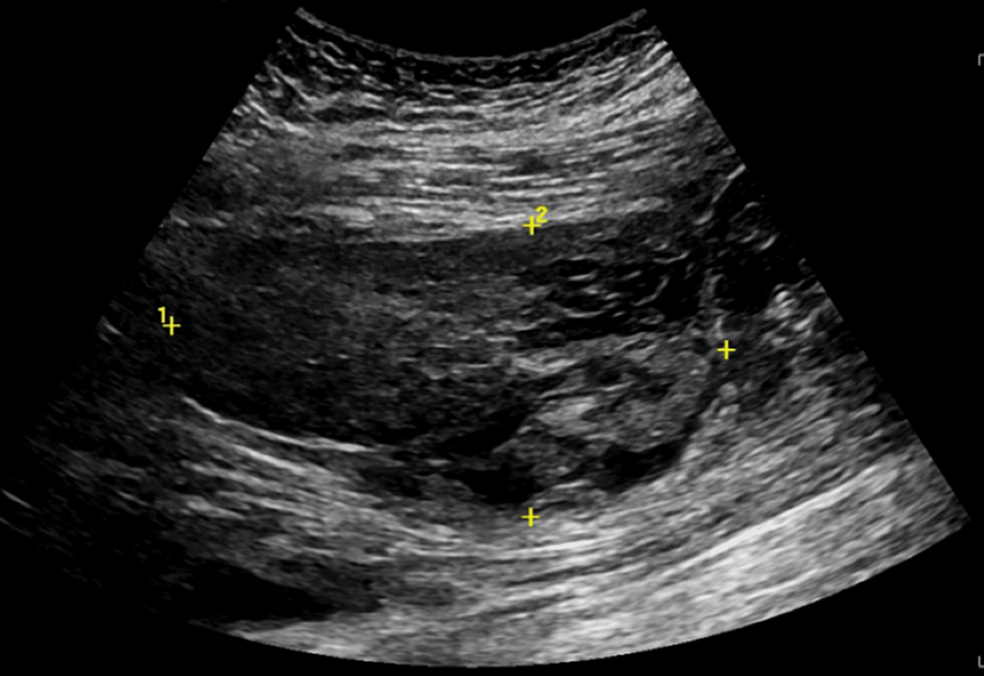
Ultrasound findings of a large hypoechogenic mass within muscle tissue with no Doppler flow are most consistent with a hematoma formation.
